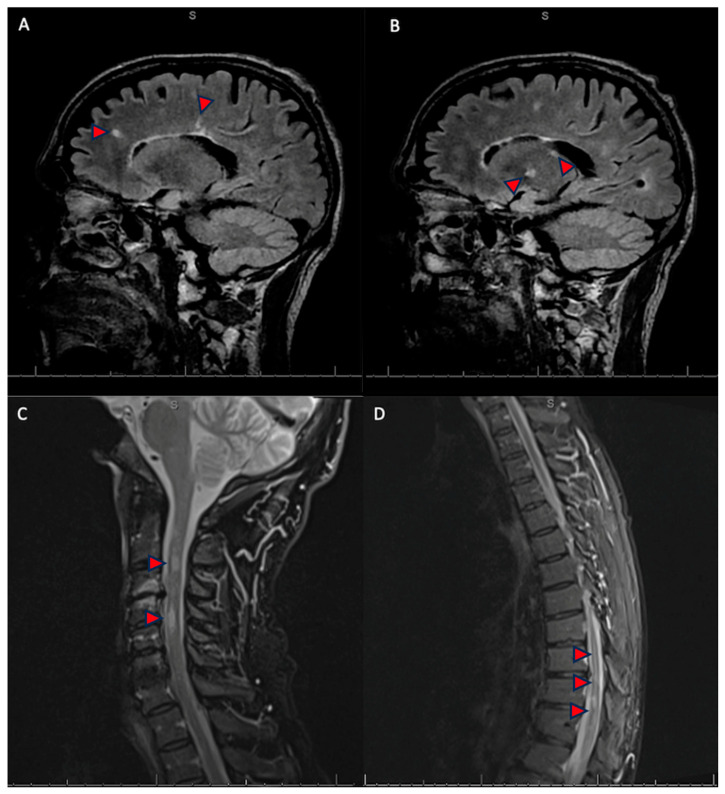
Sagittal brain MRI T2FLAIR sequence (A,B), and cervical (C) and thoracolumbar (D) MRI STIR T2 sequence. Multiple T2/FLAIR hyperintense lesions are noted at the subcortical and deep white matter of the bilateral cerebral hemispheres (A), and corpus callosum as well as in the left anterior thalamus (B). Three patchy short-segment T2 hyperintense lesions within the cervical spinal cord at C2-C3 and C4-C5 levels (C). There is diffuse volume loss of the thoracic cord with multifocal hyperintense lesions with the largest clusters at T10-T12 and extending into the conus medullaris (D). There are no enhancing lesions (A–D). The red arrows are indicate the abnormal findings described.
